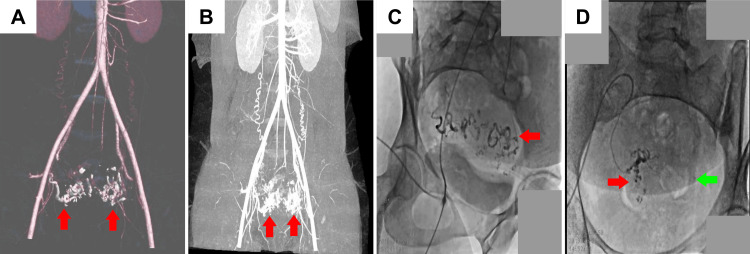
CT angiography for patient 2 (A, B) CT pelvis showing bilateral UAVM (red arrows) involving the left and right uterine wall. (C) Glue cast confirming the presence of UAVM arising from the left uterine artery. (D) Post-uterine artery embolization glue cast showing embolization of the left UAVM (green arrow) and injection of endocryl glue with lipiodol through microcatheter on the right side (red arrow). UAVM: uterine arteriovenous malformation
